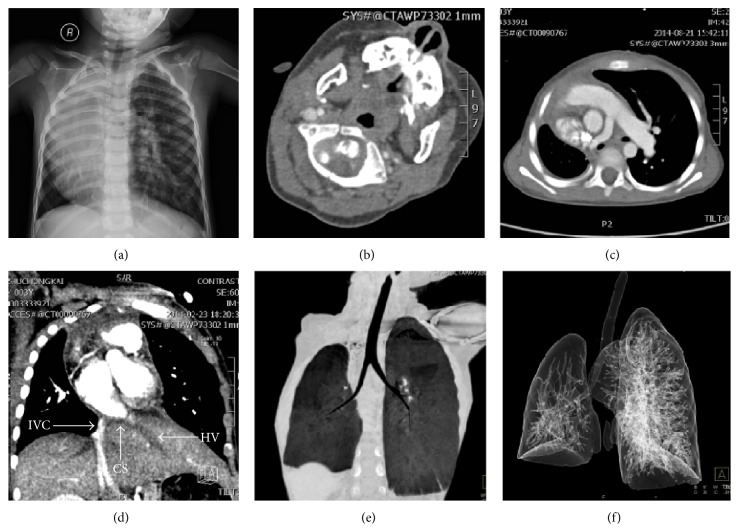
X-ray chest showing (a) a homogenous opacity occupying most of right hemithorax and compensatory hyperinflation on left side. Cranial computed tomography demonstrating (b) imperforation of right external acoustic meatus. The CECT chest in mediastinal window showing (c) right lung hypoplasia, left lung tissue crossing through the mediastinum, and hypoplastic right pulmonary artery and (d) hepatic vein that directly drains into right atrium thorough coronary sinus. CECT chest in lung window showing that (e) the right side of thoracic cage is mildly collapsed and no obvious branches of the right main bronchus could be observed. Volume-rendering computed tomography 3-dimensional reconstruction showing (f) right lung hypoplasia and a compensatory hyperplasia left lung.
